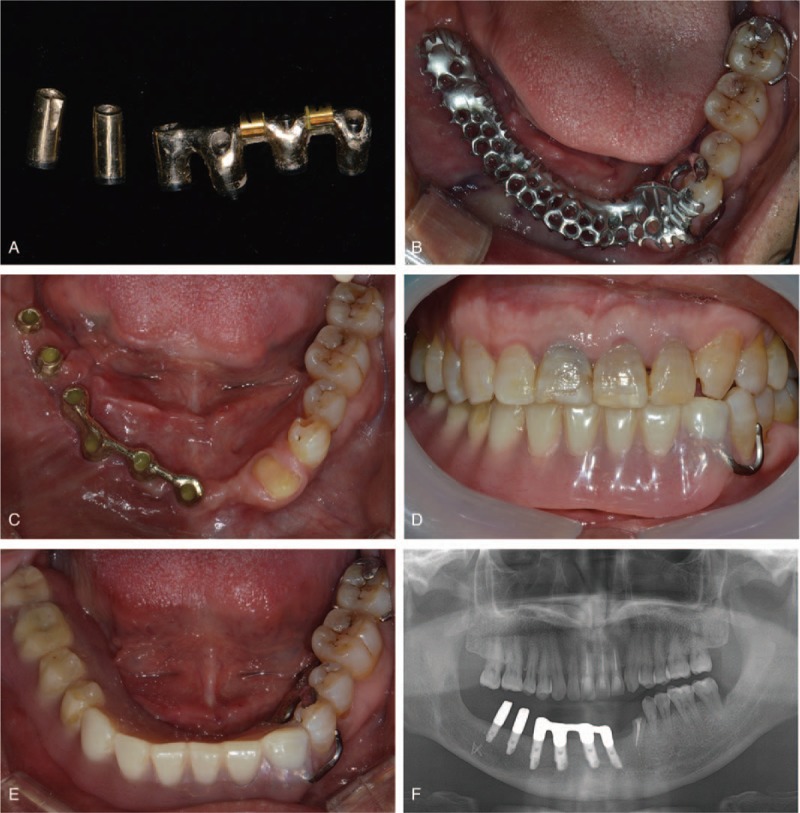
Restorative phase. (A) Casted abutments and bar attachments. (B) Metal framework try-in. (C) Occlusal view of the implant superstructures. (D) Frontal view of the definitive prosthesis. (E) Occlusal view of the implant-assisted removable partial denture (F) Panoramic radiograph at the 3-year follow-up visit.
